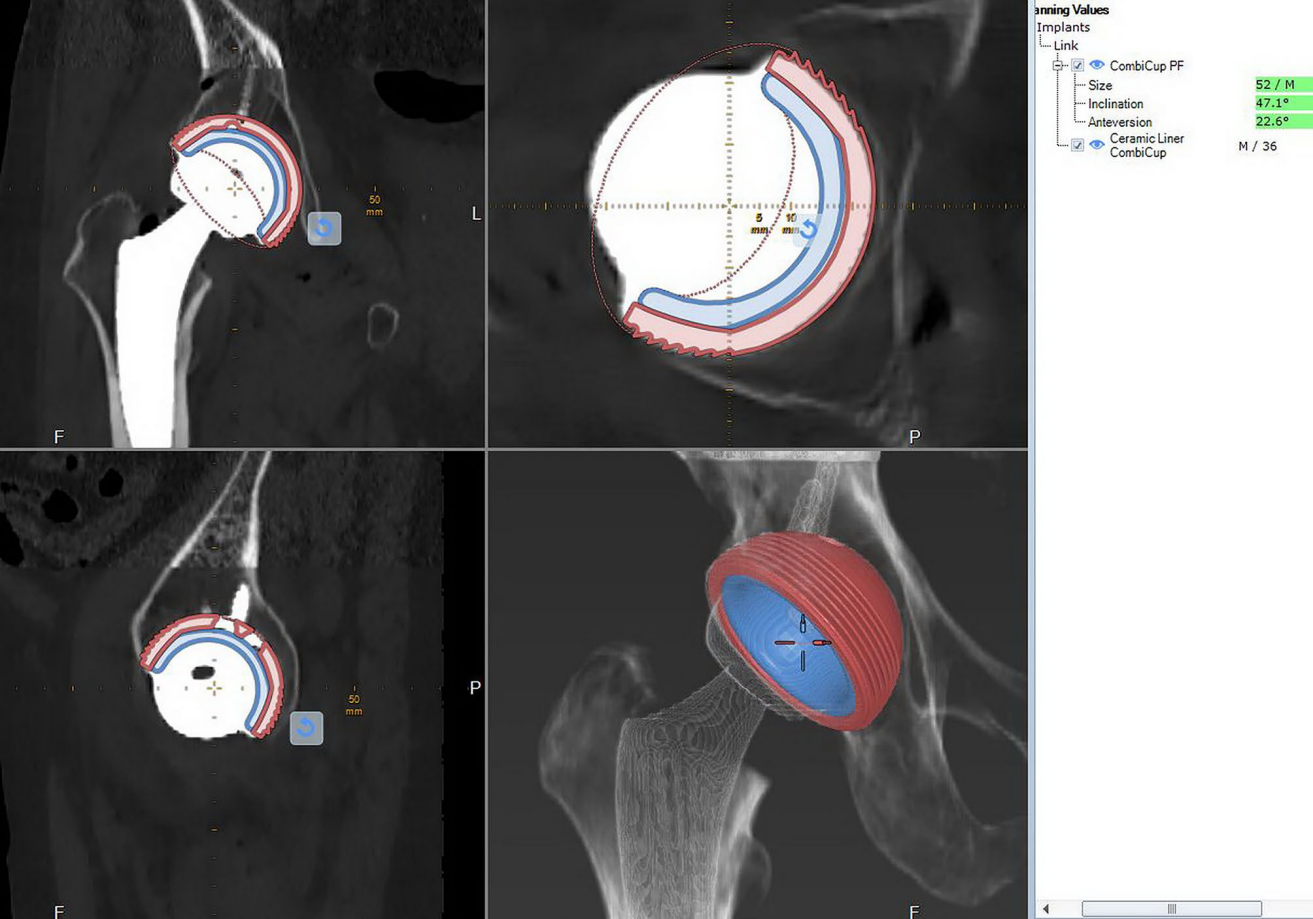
Evaluation of cup orientation on post-operative CT scan by Medicad. Using the method of overlaying the image of implant data available in the software onto the actual implant, it is possible to measure the postoperative indicators and compare them with the templating index
